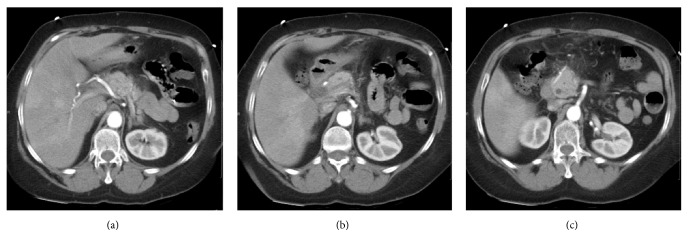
Computed tomography angiography of the chest and abdomen. Contiguous axial images demonstrate mild hazy linear increased attenuation adjacent to the neck (a) and head ((b) and (c)) of the pancreas, representing inflammatory edema.
